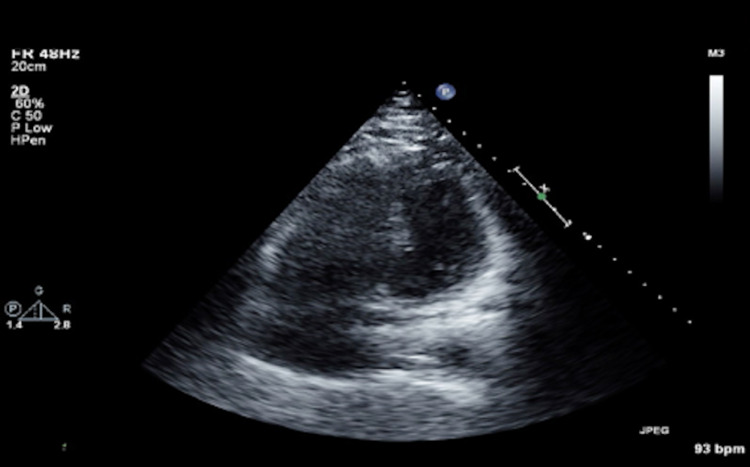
Echocardiography showing an enlarged and hypokinetic right ventricle as a direct result of the submassive pulmonary embolism
